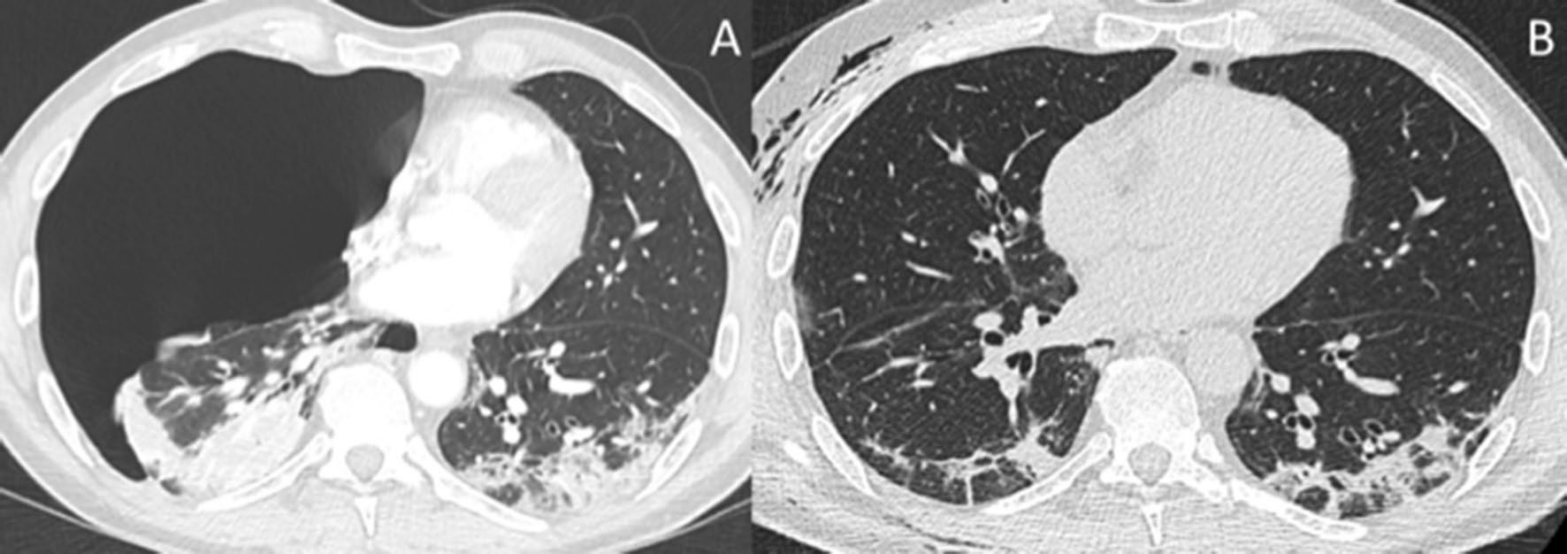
Axial CT images obtained with intravenous contrast at second admission show a right-sided tension pneumothorax with mediastinal shift to the left and right heart compression (a). Unenhanced axial CT images obtained 8 days after second admission show remission of tension pneumothorax, new soft tissue emphysema of the right chest wall and mild pneumomediastinum (b). Bilateral GGO and consolidations with peripheral distribution on both images are signs of COVID-19 pneumonia. CT, computed tomography; GGO, ground glass opacities
